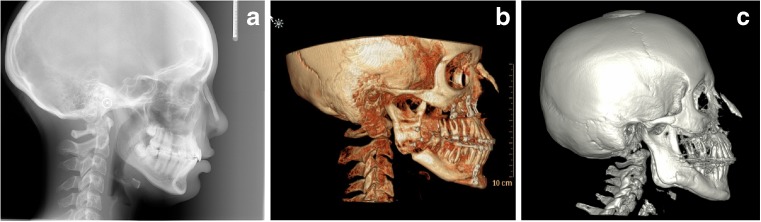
Postoperative radiographic imaging of the other three cases. a Four-month postoperative lateral cephalogram. b Six-month postoperative CBCT scan. c One-year postoperative CBCT scan
